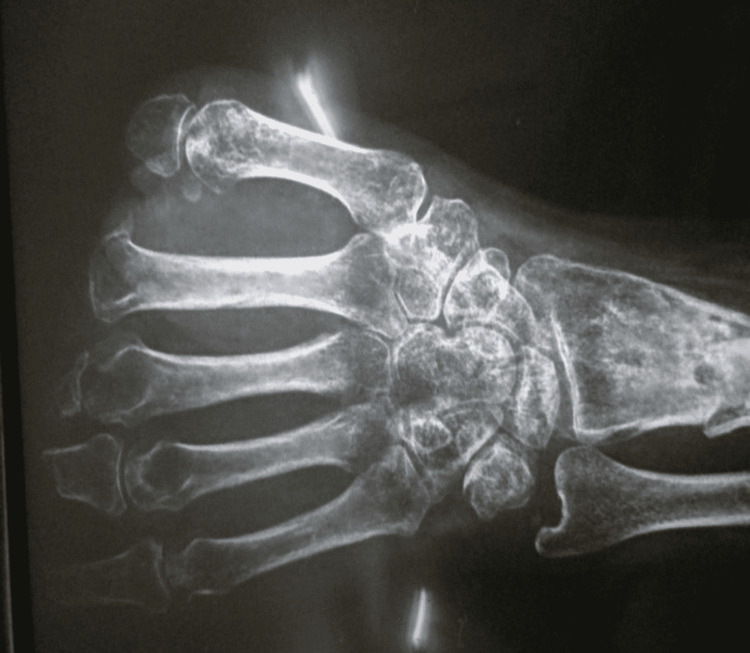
Radiograph of the left hand depicting the level of amputations.
